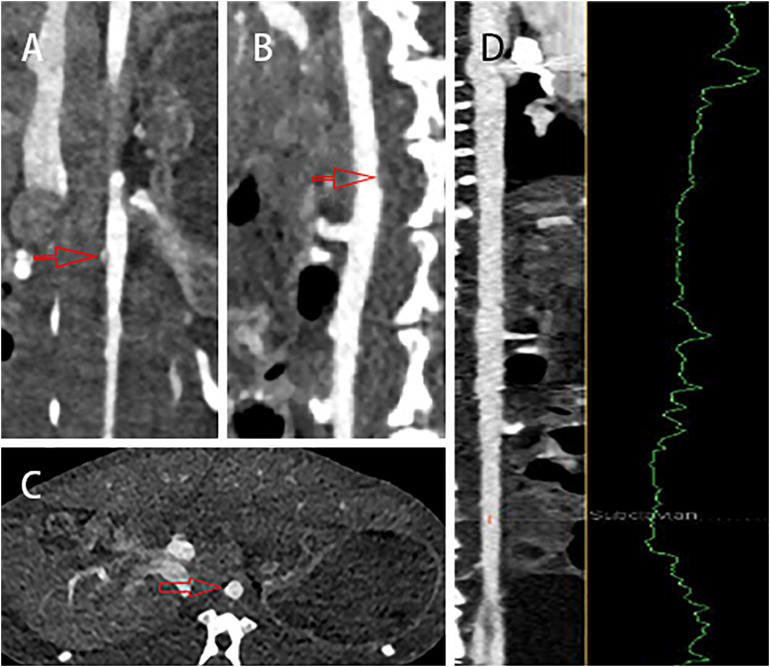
The (A) coronal, (B) sagittal, and (C) cross-sectional CT scans showed that the wall of abdominal aorta was thickened and irregular (red arrow), but there was no obvious sign of atherosclerotic plaques. (D) In addition, there was no significant stenosis in the whole abdominal aorta.
